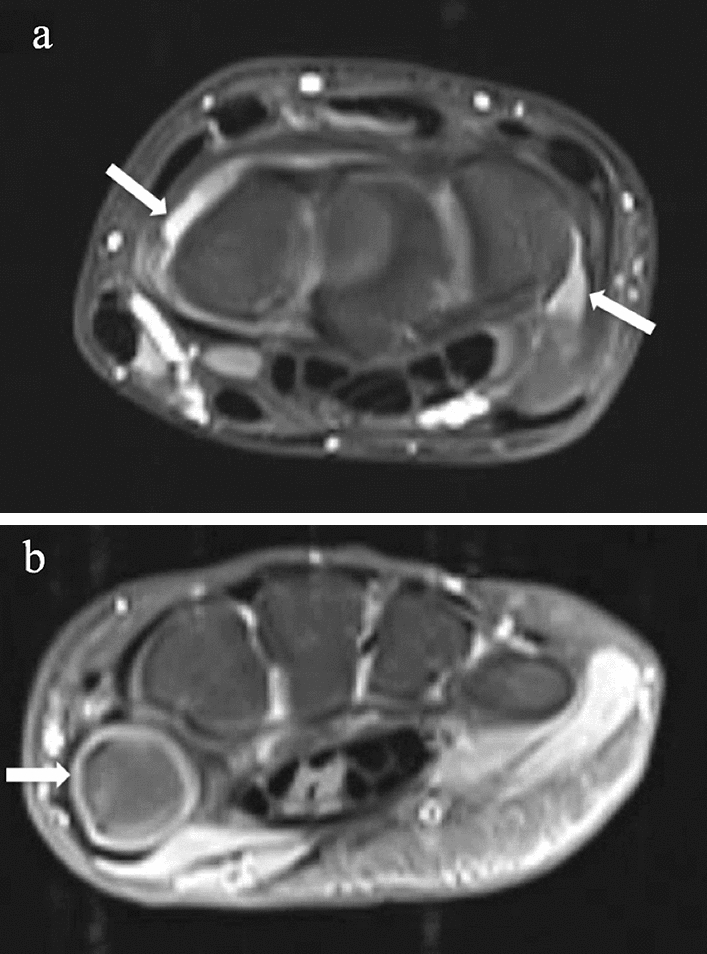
Hyper-intense cartilage mimics synovial enhancement. T1-weighted contrast-enhanced and fat-saturated MRI of a moderately increased synovial enhancement in the wrist of a 15-year female patient indicated by the arrows and b hyper-intense cartilage of the wrist in a 10-year old female patient indicated by the arrow
